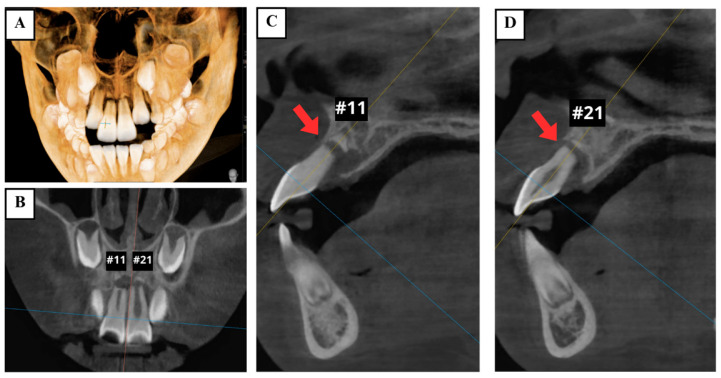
Preoperative CBCT images showing horizontal root fractures in the apical third of two maxillary central incisors (arrows). (A) 3D reconstruction; (B) coronal view; (C) sagittal views of the right maxillary central incisor (tooth #11); and (D) left maxillary central incisor (tooth #21).
